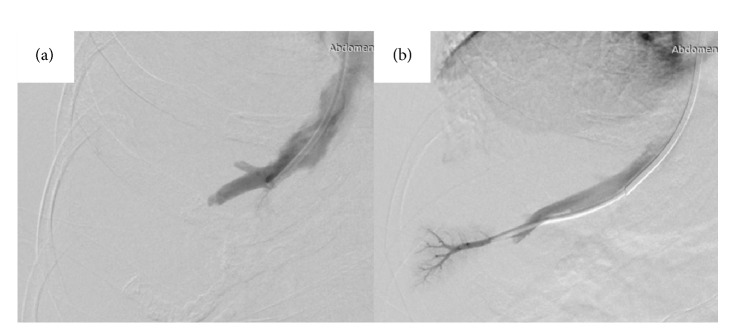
Transjugular liver biopsy was performed under X-ray fluoroscopy guidance. (a) A venogram showing a 5-Fr catheter inserted into the right hepatic vein. (b) A postbiopsy venogram, showing no leakage of contrast agent to the outside of the liver.
